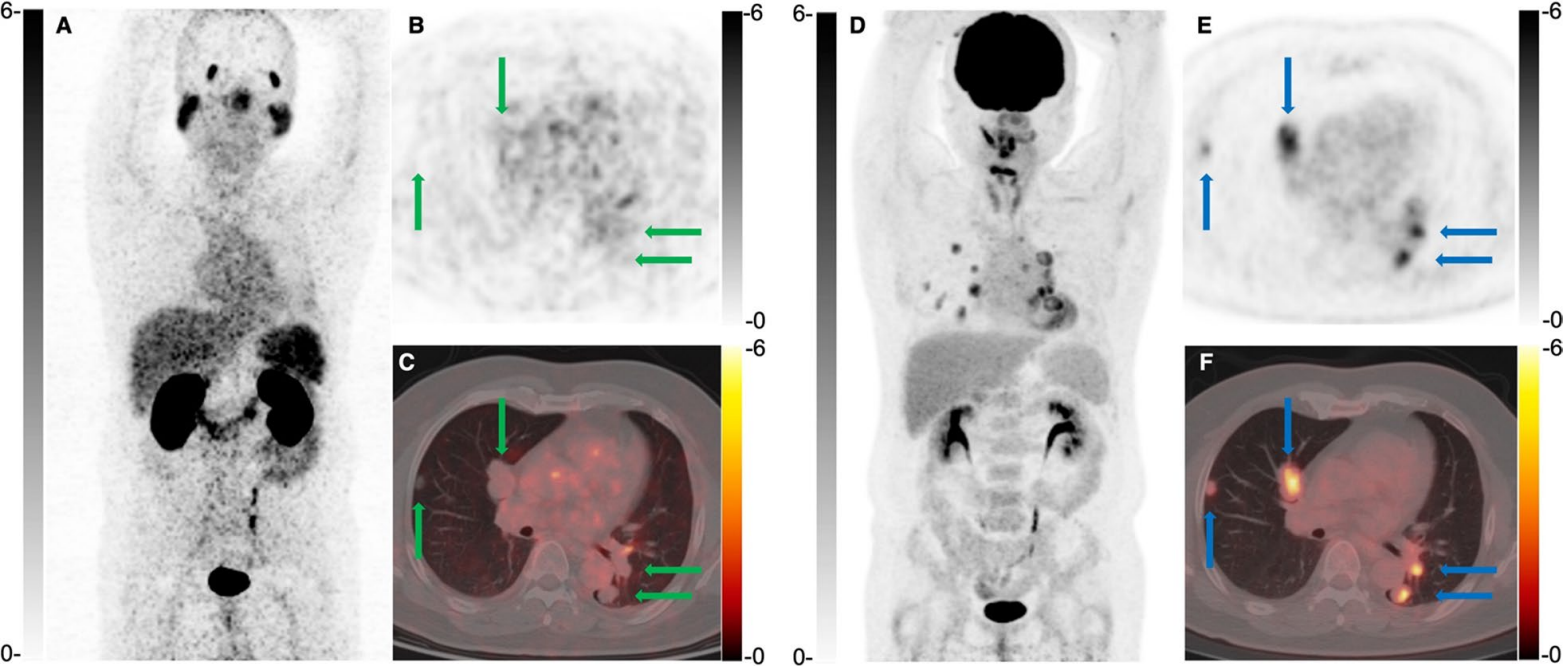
A 49-year-old man was diagnosed with local recurrence and distant metastases 74 months after surgical removal of a left maxillary ACC. ^68^ Ga-PSMA PET/CT (A–C) showed multiple lung metastases with negligible uptake (green arrow). ^18^F-FDG PET/CT (D–F) revealed FDG-avid pulmonary lesions (blue arrow, SUVmax 7.1)
